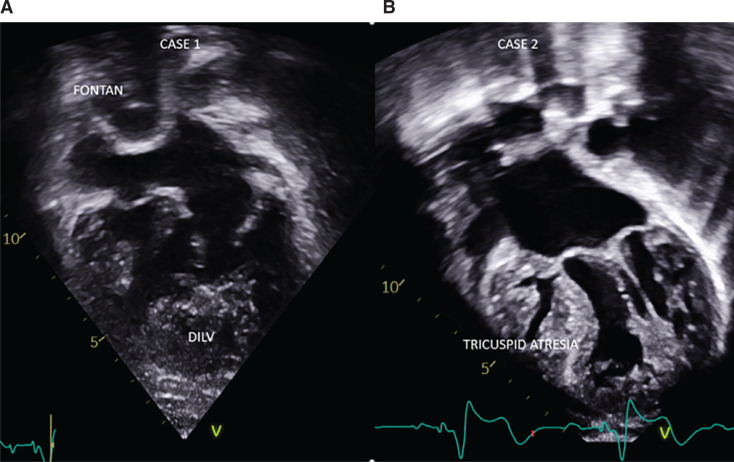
Echocardiographic views demonstrating single ventricular anatomy of the two Fontan cases. A: Case 1 with double inlet left ventricle (status post–lateral tunnel Fontan). B: Case 2 (right image) with tricuspid atresia and hypoplastic right ventricle in a patient with heterotaxy syndrome (left atrial isomerism, interrupted inferior vena cava with azygous continuation).
